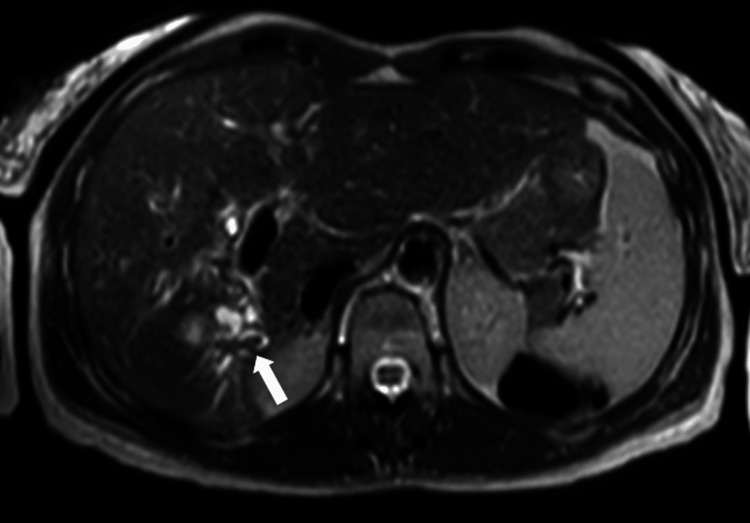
Axial T2-weighted MRI image of the liver Axial T2-weighted MRI showing biliary casts that appear isotense to hypointense relative to bile (white arrow).
